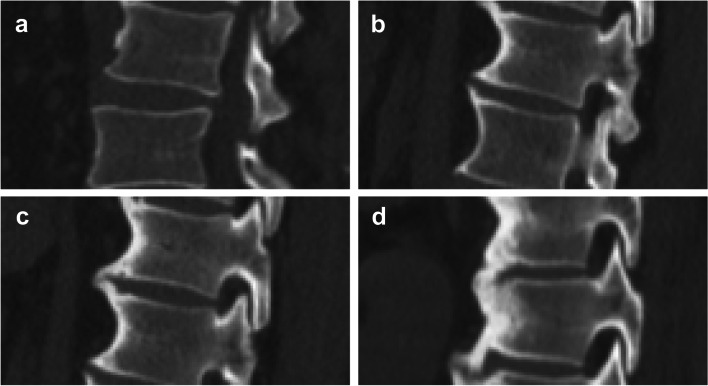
The bridge scores were evaluated in sagittal CT image. A score from 0 to 3 was assigned for each vertebral segment. a: A score of 0 indicates normal vertebral bodies without formation of new bone; b: A score of 1, anterior new bone formation without a solid bony bridge OR a connection between two adjacent vertebral bodies without abundantly formed bone;c: A score of 2, near complete bridging by the anterior new bone formation with less than 2 mm of distance between the bony structures or a full connection of the bone in a maximum of two sagittal or coronal CT sections; d: A score of 3, complete bridging between the vertebral bodies above and below the disc with abundant new bone formation in more than two sagittal or coronal CT section
